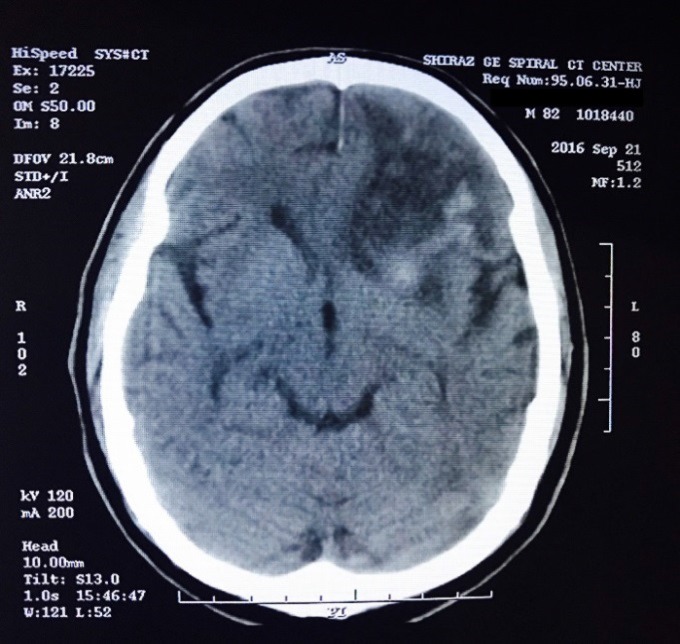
Brain computerized tomography (CT) scan indicating a hemorrhage in the left frontoparietal lobe
